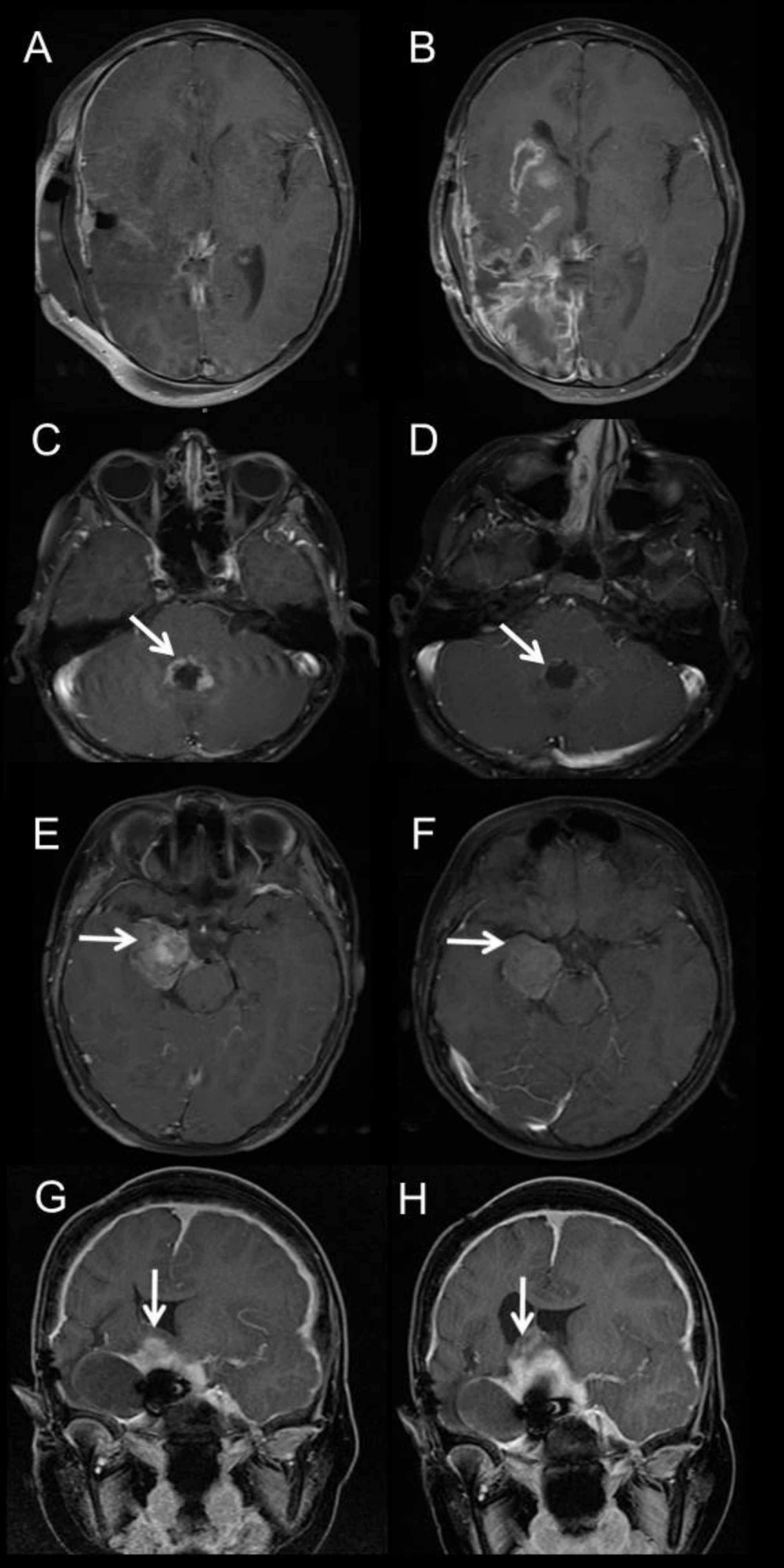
Magnetic resonance imaging findings pre- and post-targeted therapy A: Pre-targeted treatment (palbociclib and trametinib) MRI on a three-year-old boy with high-grade glioma (amplification of CCND2, CDK4, and MDM2 amplification). B: Post-treatment MRI showing progressive disease one month after therapy. C: Pre-treatment (everolimus and trametinib) MRI in a 10-year-old male with NF1 and multiply recurrent PDGFRA-mutated juvenile pilocytic astrocytoma of the posterior fossa (arrow). D: Post-treatment MRI reveals a near-complete response of the fourth ventricular tumor (arrow). E: Pre-treatment (vemurafenib) MRI in a four-year-old boy with V600E-mutated pleomorphic xanthoastrocytoma of the right temporal lobe (arrow). F: Post-treatment MRI reveals partial response (arrow). G: Pre-treatment (trametinib) MRI in a 17-year-old male with a GLI 1, P53-mutant multiply recurrent suprasellar low-grade glioma (arrow). H: Post-treatment MRI reveals stable suprasellar disease (arrow). MRI, magnetic resonance imaging
